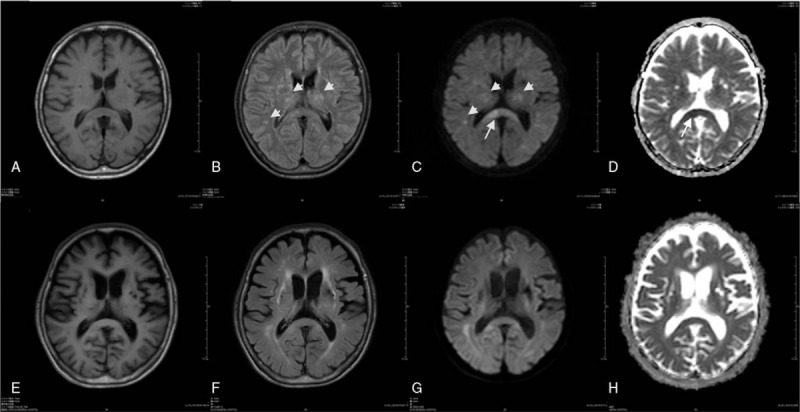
Brain MRI on the eighth hospital day. (A) T1 weighed image (T1WI); (B) fluid attenuated inversion recovery (FLAIR); (C) diffusion weighted image (DWI); (D) apparent diffusion coefficient (ADC) map) and 7 months after symptom onset; (E) T1WI; (F) FLAIR; (G) DWI; (H) ADC map. On acute phase, the splenium of the corpus callosum (SCC) lesion appeared DWI high intensity with low ADC (arrow). Lesions in bilateral thalami and cerebral white matter (arrowhead) showed high intensity in FLAIR and high intensity in DWI with low ADC. After 7 months, the SCC lesion disappeared but cerebral atrophy progressed. ADC = apparent diffusion coefficient, DWI = diffusion weighted image, FLAIR = fluid attenuated inversion recovery SCC = splenium of the corpus callosum, T1WI = T1 weighed image.
